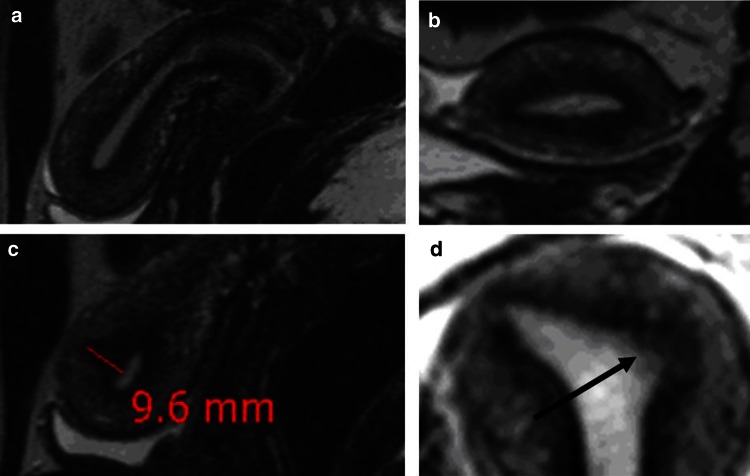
MRI scans of a 25 years old patient with extreme primary dysmenorrhea as an example for the establishment of the diagnosis of adenomyosis by ‘visualisation’. The diameter of the JZ in the mid-sagittal plane would not allow for the diagnosis of adenomyosis (a). A closer analysis of an enlargement of the JZ in the left cornual angle (b and c) revealed beginning cystic cornual angle adenomyosis (d) (arrow)
